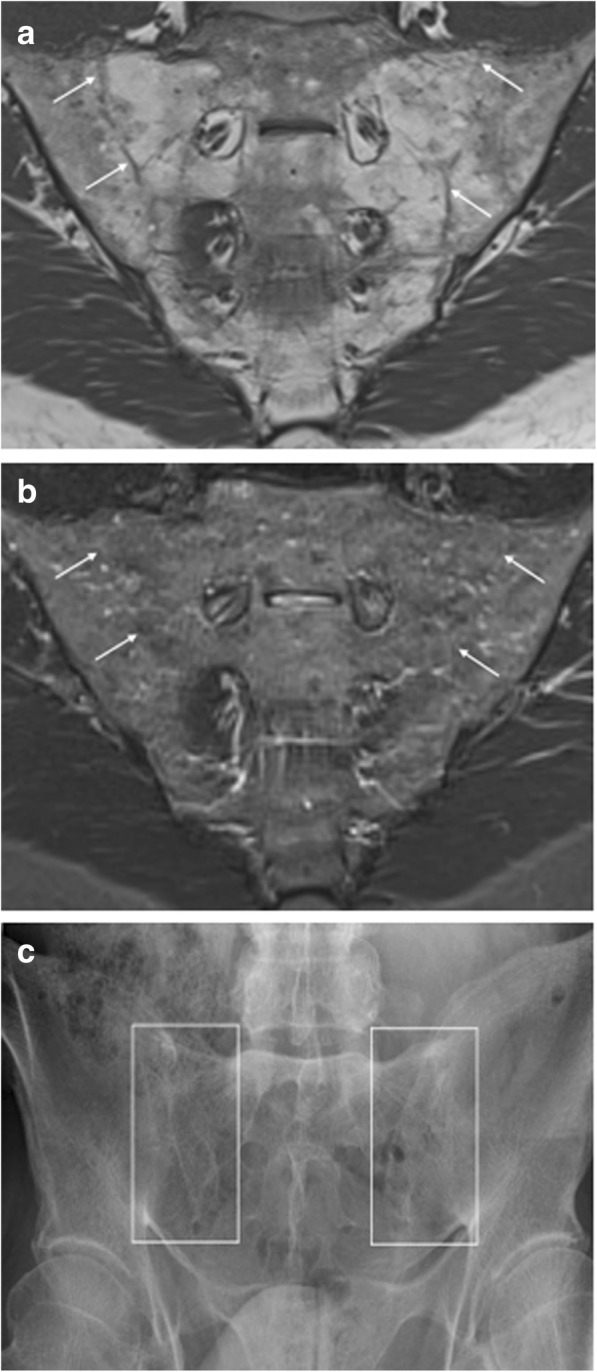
Ankylosis of the sacroiliac joints. Coronal oblique MR images acquired in a 53-year-old man with SpA show bony fusion of the sacroiliac joints as (a) high signal intensity on T1-weighted imaging (fatty degeneration) and (b) low signal intensity on STIR imaging. A sclerotic remnant of the sacroiliac joints is highlighted (arrows). c Radiography of the sacroiliac joints confirms these structural bony changes
